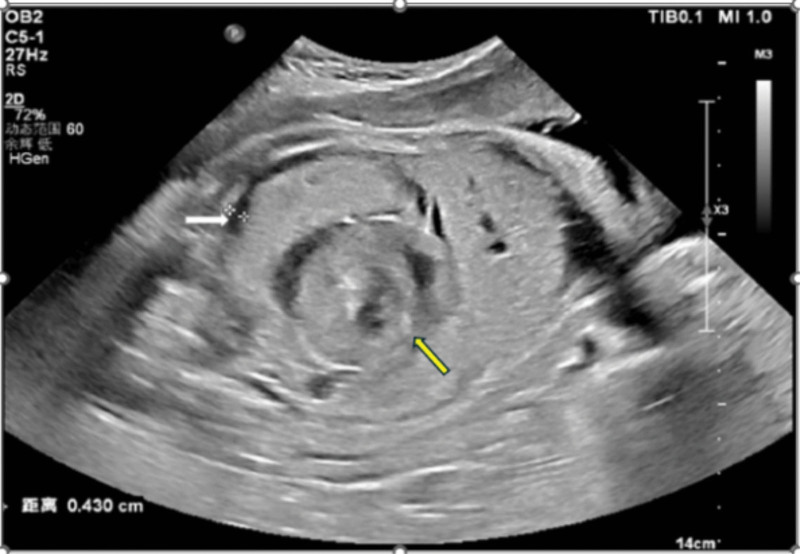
Fetal ultrasound revealed intestinal dilation with ascites (white arrow), Whirlpool sign (yellow arrow).
